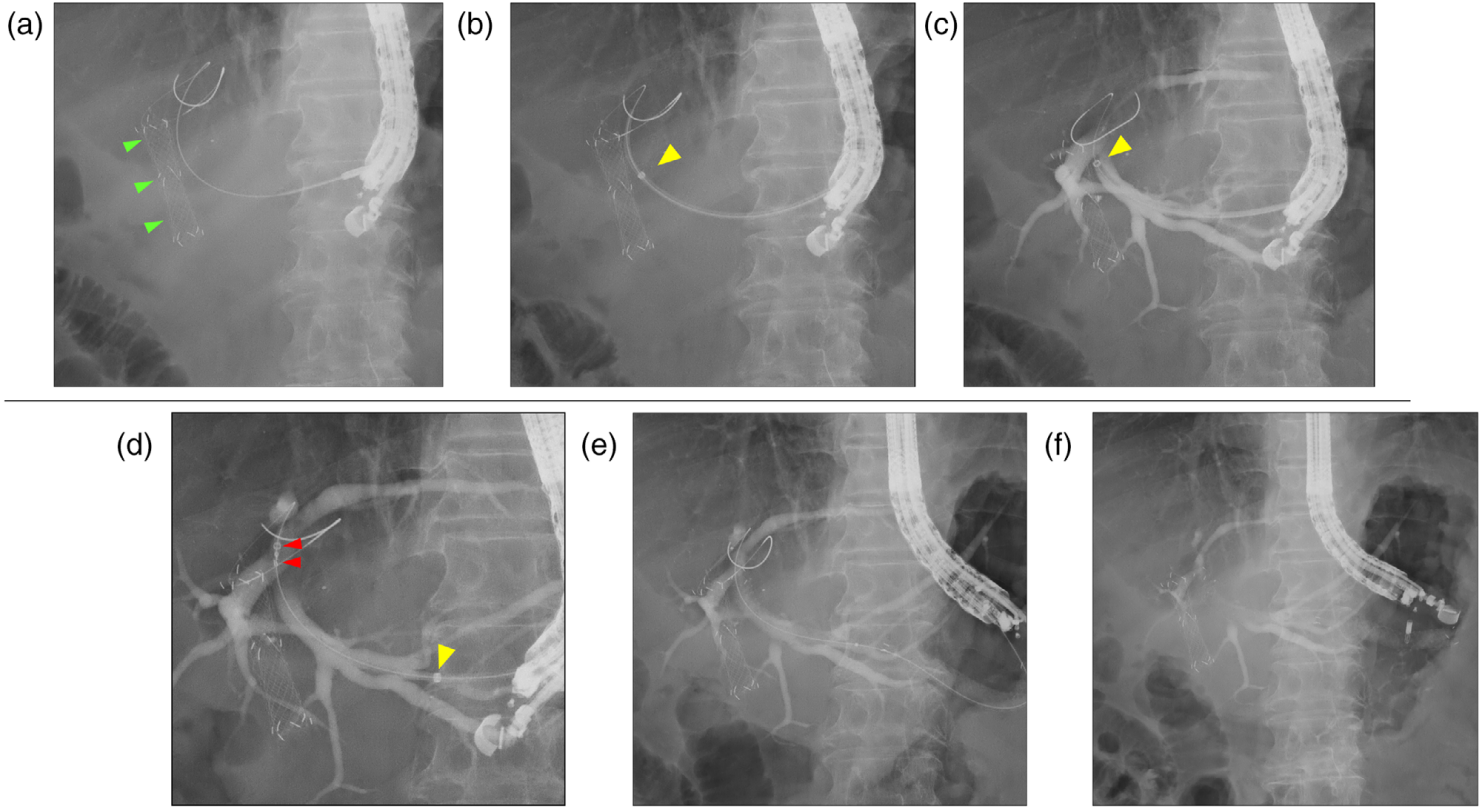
Fluoroscopic cholangiogram showing the stenting technique using the tapered sheath dilator (EndoSheather) in endoscopic ultrasound‐guided hepaticogastrostomy (EUS‐HGS). Green arrowheads indicate the deployed stent under endoscopic retrograde cholangiopancreatography. (a) Proper insertion of a guidewire. (b) Utilization of the tapered sheath dilator to mechanically dilate the needle tract. Yellow arrowhead indicates the radiopaque marker of the outer sheath. (c) Removal of the inner catheter, leaving the outer sheath in position within the bile duct. The outer sheath enabled aspiration of bile juice and injection of contrast medium. (d–f) Smooth insertion and deployment of a fully covered self‐expandable metal stent with a 5.9 Fr delivery system. Red arrowheads show the markers of the metal stent.
